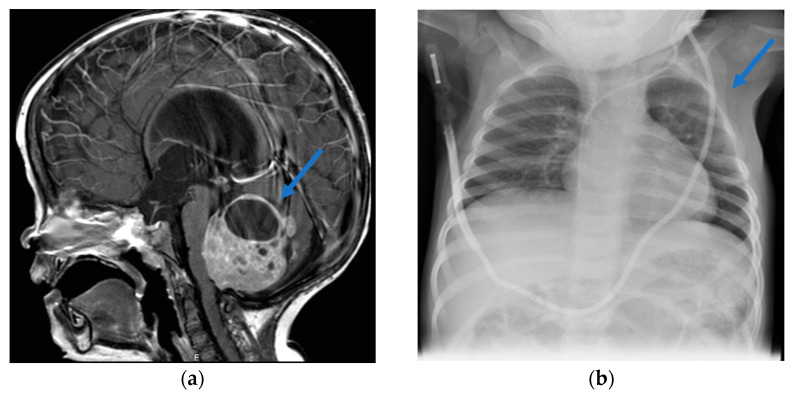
Clinical features of the patient at admission to our Institution. (a) Sagittal T1-weighted 3D Turbo Field Echo (TFE) contrast enhanced brain Magnetic Resonance Imaging (MRI) shows an expansive lesion involving the IV ventricle and the cerebellar vermis (blue arrow) and causing hydrocephalus. (b) Chest X-ray, showing a bifid rib (blue arrow) with an incomplete fusion of the III and IV left ribs.
